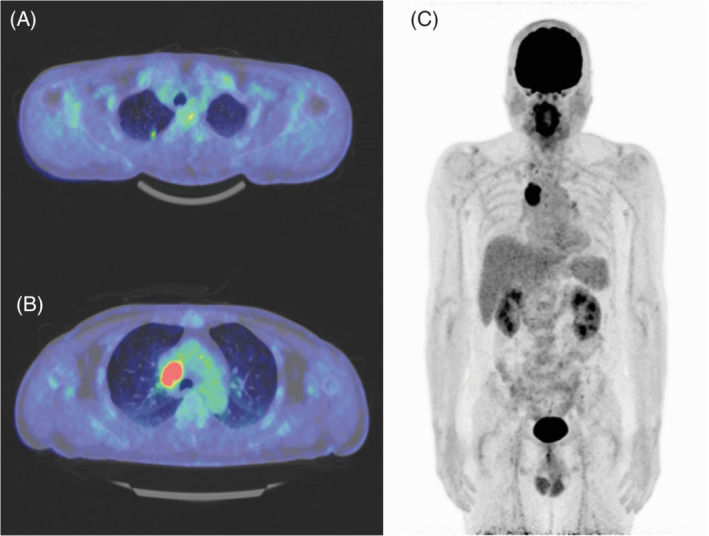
Positron emission tomography‐computed tomography. (A). A nodular shadow was found in the right lung apex, with fluorodeoxyglucose (FDG) accumulation with a maximum standardized uptake value (SUV_max_) of 2.5. A benign nodule, such as inflammatory granuloma, was suspected. (B) The right tracheobronchial lymph node was swollen, with FDG accumulation with an SUV_max_ of 10.7. The density inside the lymph nodes and their margins were smooth, suggesting lymphoproliferative disease. (C) There was no other abnormal uptake of FDG except the above two lesions
